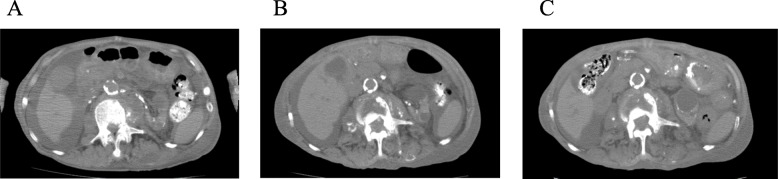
Computed tomography showed the gradual reduction of the left psoas abscess. a Day 5, the day after meropenem and vancomycin were initiated, b day 49, and c day 88
